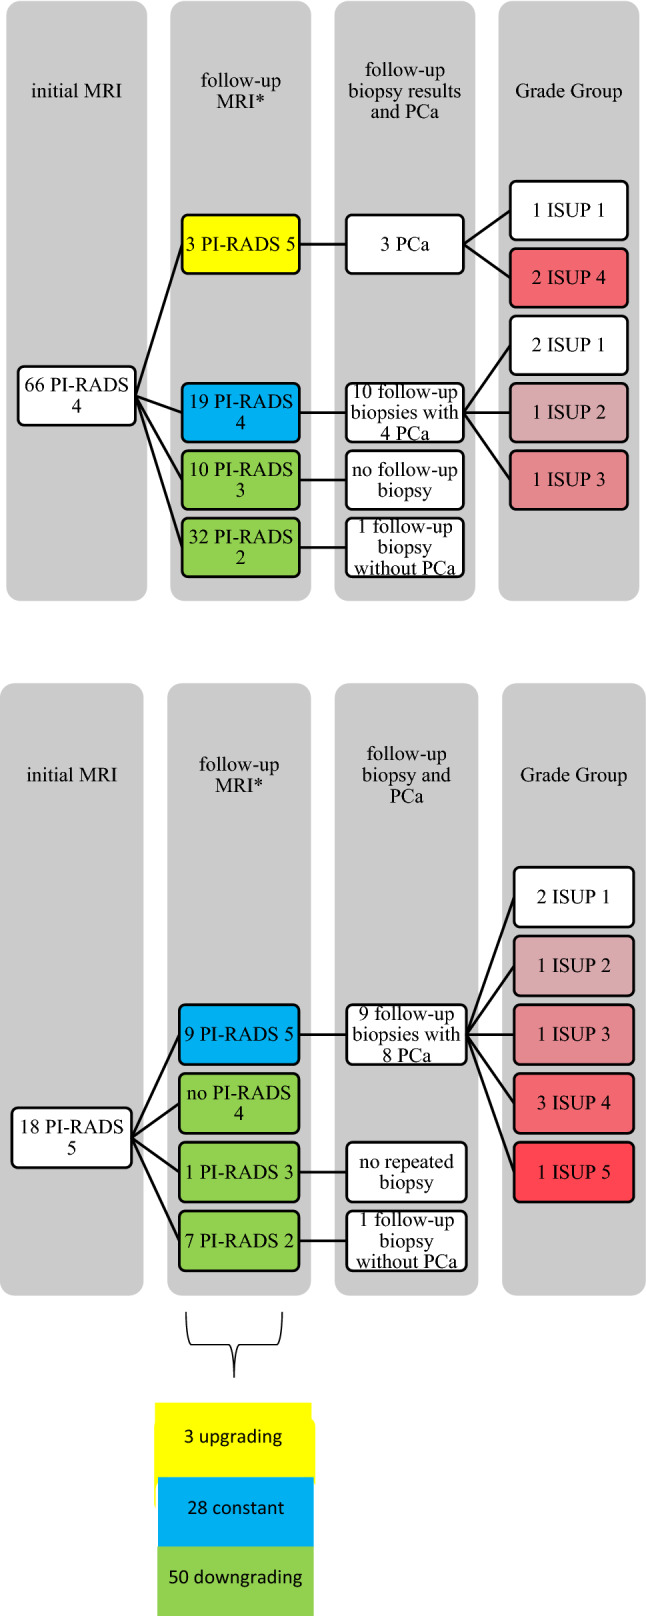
Relation of initial MRI and follow-up MRI, the allocation to a follow-up biopsy and the resulting ISUP Grade. (*on follow-up three PI-RADS Scores unknown).
